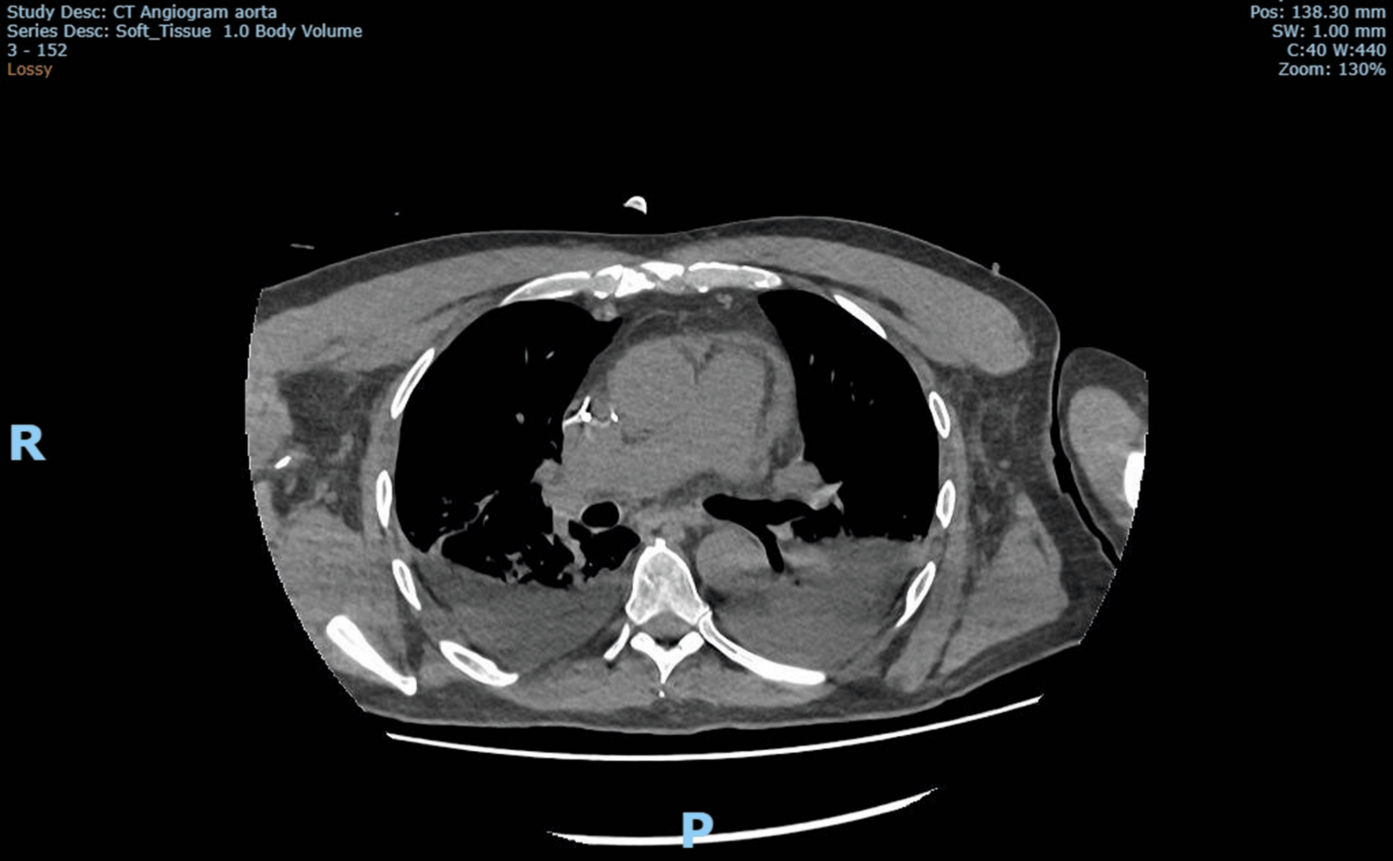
CT aortogram Pos: position, SW: section width, R: right, P: posterior.
